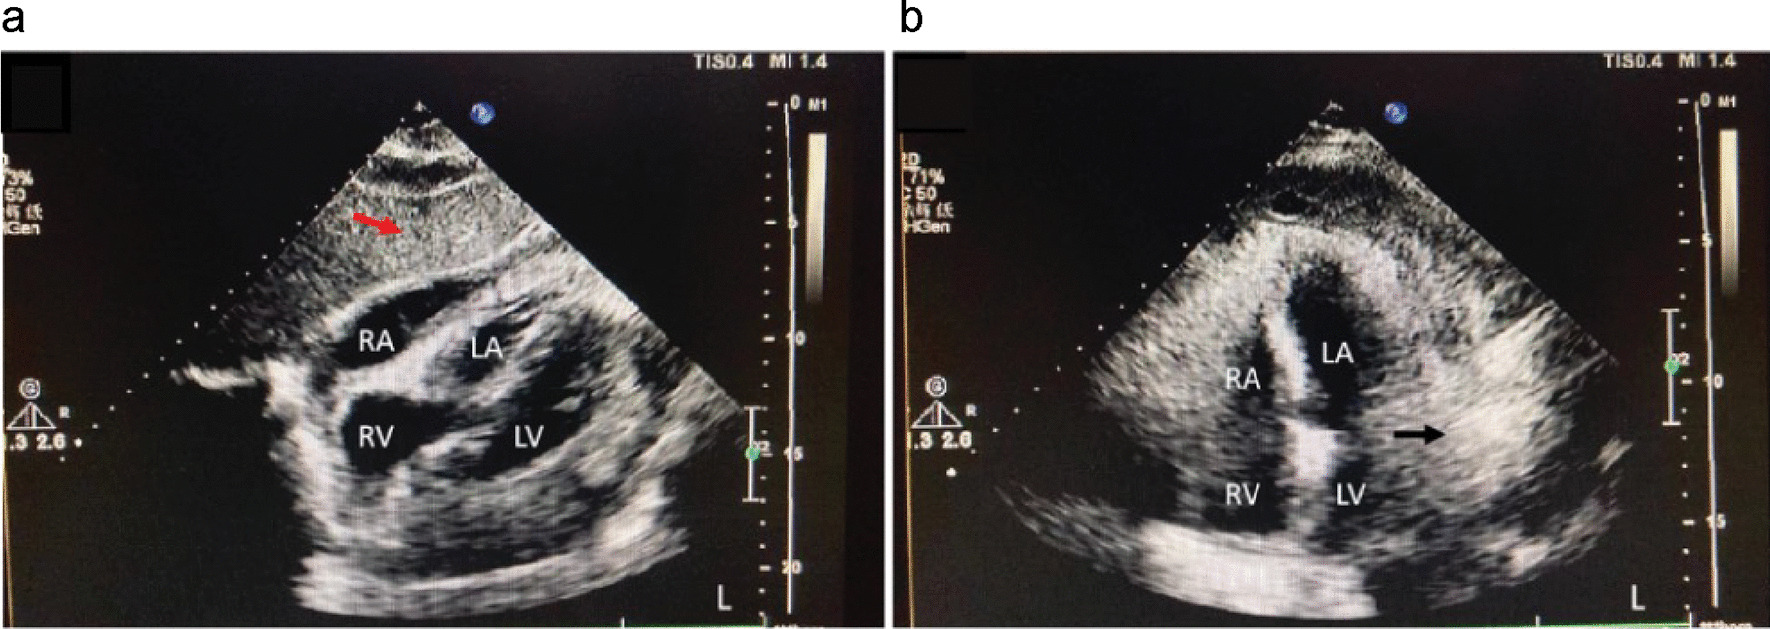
Transthoracic echocardiography. Transthoracic echocardiograph revealing thickening of the anterior pericardial wall (A red arrow) and uneven hyperechoic masses extending to the pericardial cavity from the pericardium along the lateral wall of the left ventricle (B black arrow)
